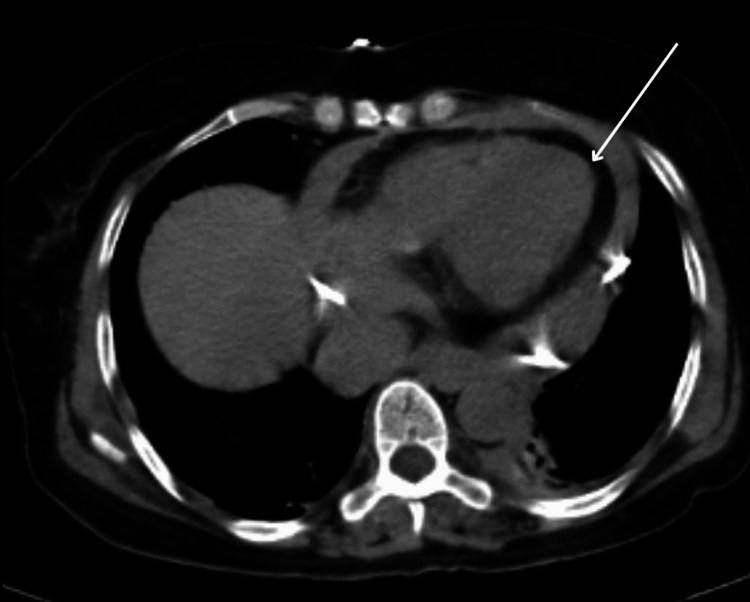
High-resolution CT showing pericardial effusion, indicated by the white arrow CT, computed tomography
